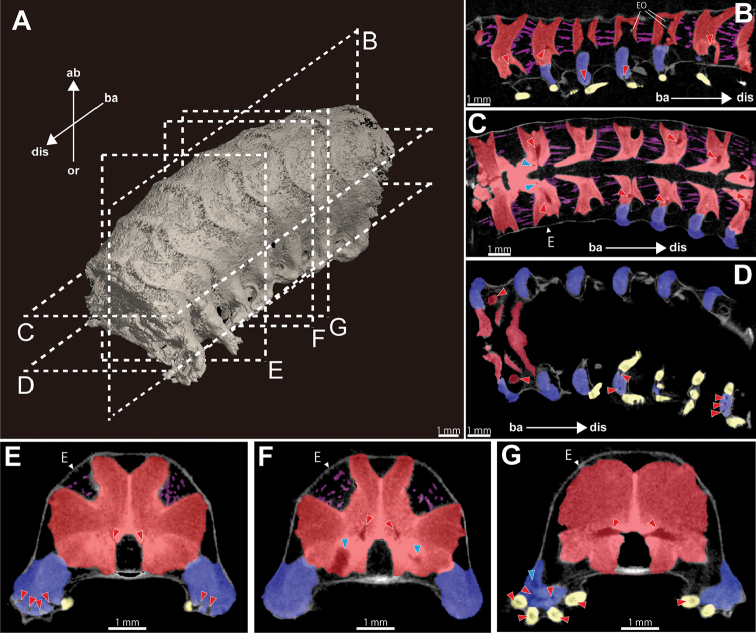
Micro CT surface rendered (A) and 2D section (B–G) images of the basal part of arm (7–13th arm segments) of *Asteronyx
